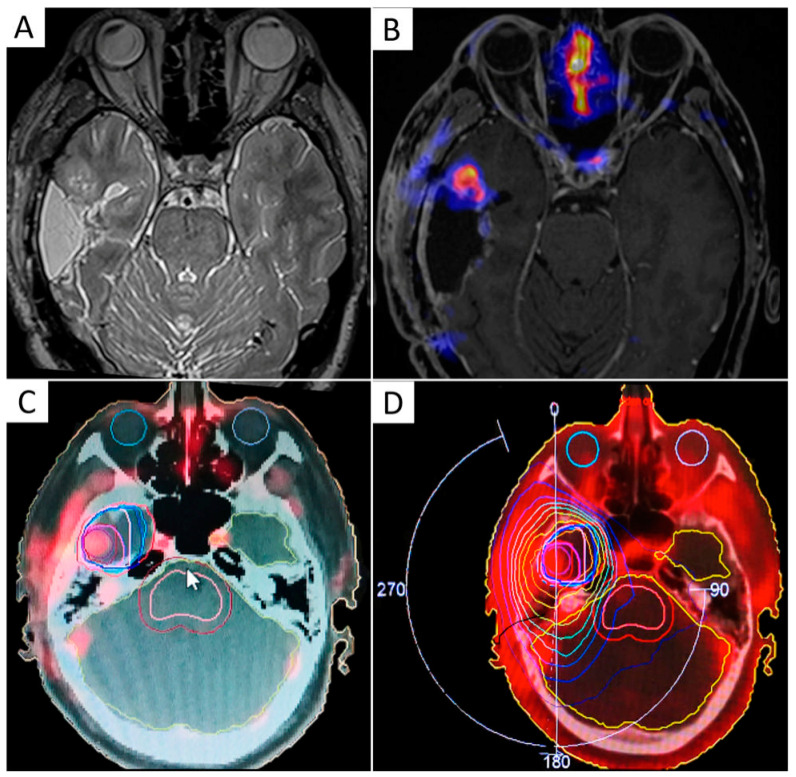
Example of a volumetric modulated arc therapy (VMAT) sequential boost of radiation to hypoxia-positive recurrent GBM. T2-weighted and contrast-enhanced T1-weighted MRI sequences were acquired along with ^64^Cu-diacetyl-bis(N^4^-methylthiosemicarbazone) (ATSM) PET on a hybrid 3T PET/MR scanner, three hours after radiopharmaceutical injection (A,B). 37.5 Gy were delivered in 15 daily fractions to the surgical cavity followed by a boost of radiation (5 Gy) to the ^64^Cu-ATSM-positive tumor region, indicating chronic hypoxia (C,D).
